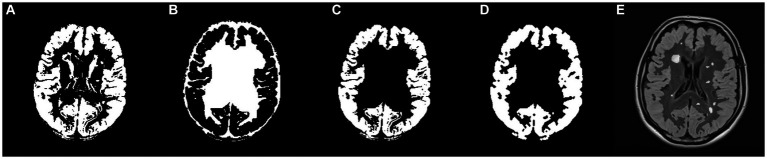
(A) Post-processed GM mask, (B) the union mask generated from WM, CSF, WMH, and ventricles masks, (C) filtered GM mask by the union mask, (D) post-processed, filtered GM mask, and (E) FLAIR image with the imposition of the generated GM mask and highlighted WMH.
